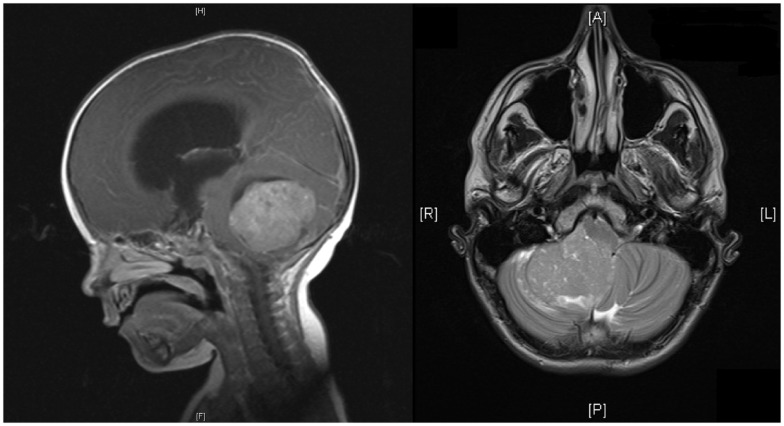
MRI of head showing sagittal and horizontal views. Sagittal view shows a midline posterior fossa medulloblastoma with intermediate signal intensity. There is an obstruction to the flow of CSF, marked hydrocephalus, and edema. Horizontal view shows a homogenous enhancing medulloblastoma arising from the right cerebellar hemisphere with displacement of the vermis.
